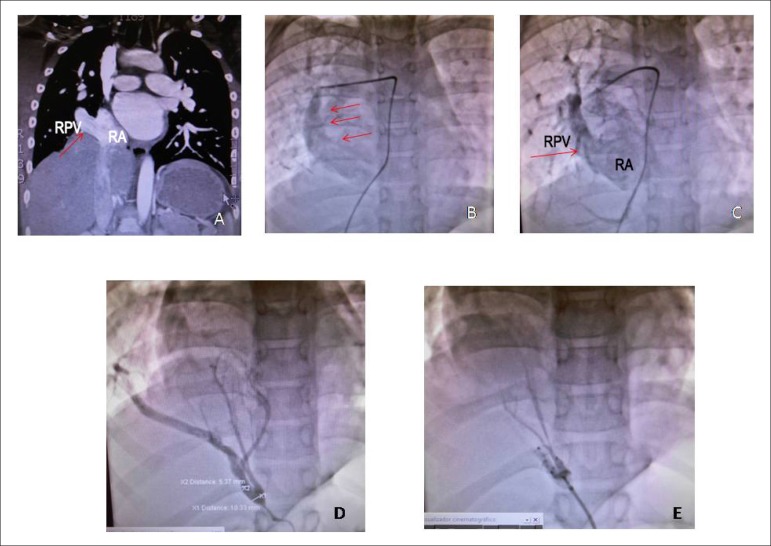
CT angiography in A showing the right pulmonary vein (RPV, arrow)
